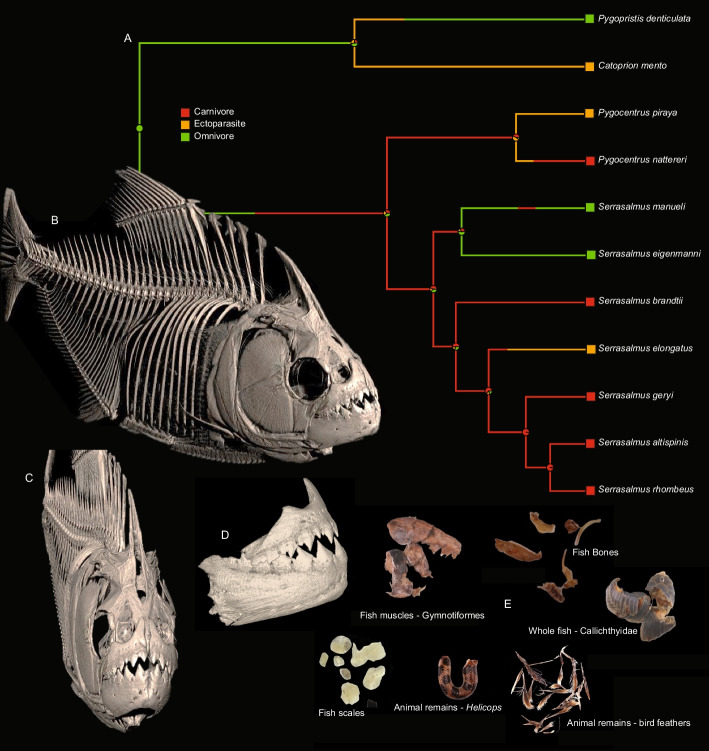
Skeletal and dental anatomy of a black piranha, *Serrasalmus rhombeus*, (ANSP 195066) obtained through micro-computed tomography data. A Ancestral state estimation of diet evolution in piranhas using stochastic character mapping. Based on diet information and phylogeny from Kolmann et al. 2021. B Lateral view of black piranha whole body. C Anterior view of black piranha. D Lateral view of the jaws. E Gut contents showing dismembered food remains (photos: D. Hemraj-Naraine). Osteological images rendered with CTVox (Bruker Softwares) and 3DSlicer (Kikinis et al. 2014). Scan parameters: Bruker SkyScan 1173, 35um, 70kV 114uA, 1 mm Al filter
